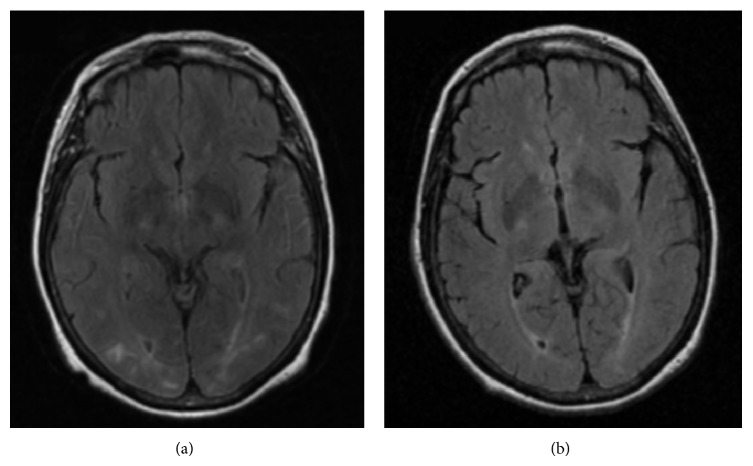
FLAIR MRI. (a) Subcortical lesions mainly located in posterior regions. (b) A month later an improvement of white matter lesions was observed.
